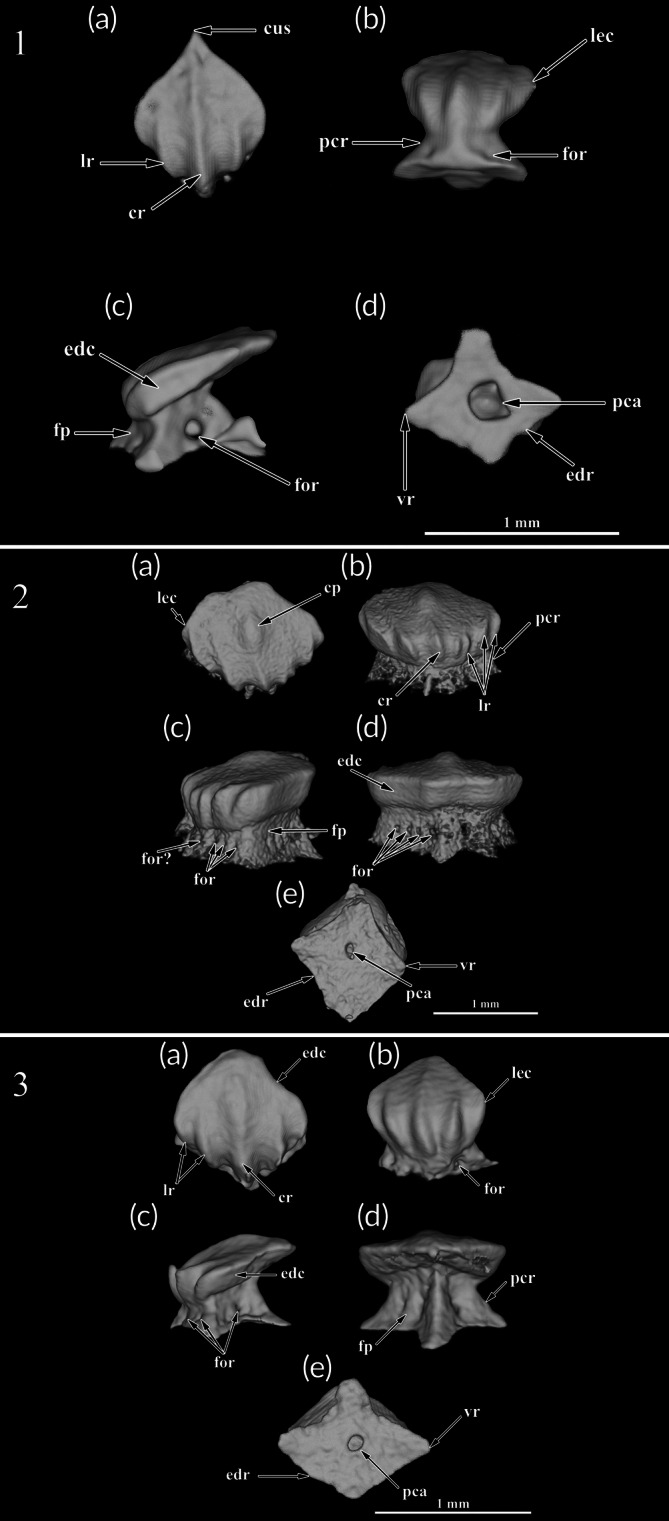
1 = Fin type 4 denticles (UFPB 14343 female, 1068 mm PCR). (1a) F6 dorsal view. (1b) F6 frontal view. (1c) F6 lateral view. (1d) F6 ventral view. In (1a, 1d) anterior to bottom; In (1b) anterior to front; In (1c) anterior to left. 2 = Fin type 5 denticles (UFPB 14343 female, 1068 mm PCR). (2a) F3 dorsal view. (2b) F3 frontal view. (2c) F3 lateral view. (2d) Posterior view. (2E) F3 ventral view. In (2a, 2e) anterior to bottom; In (2b) anterior to front; In (2c) anterior to left; In (2d) anterior to back. 3 = Fin type 6 denticles (UFPB 14343 female, 1068 mm PCR). (3a) F4 dorsal view. (3b) F7 frontal view. (3c) F4 lateral view. (3d) F8 posterior view. (3e) F7 ventral view. In (3a, 3e) anterior to bottom; In (3b) anterior to front; In (3c) anterior to left; In (3d) anterior to back (micro CT‐scan).
